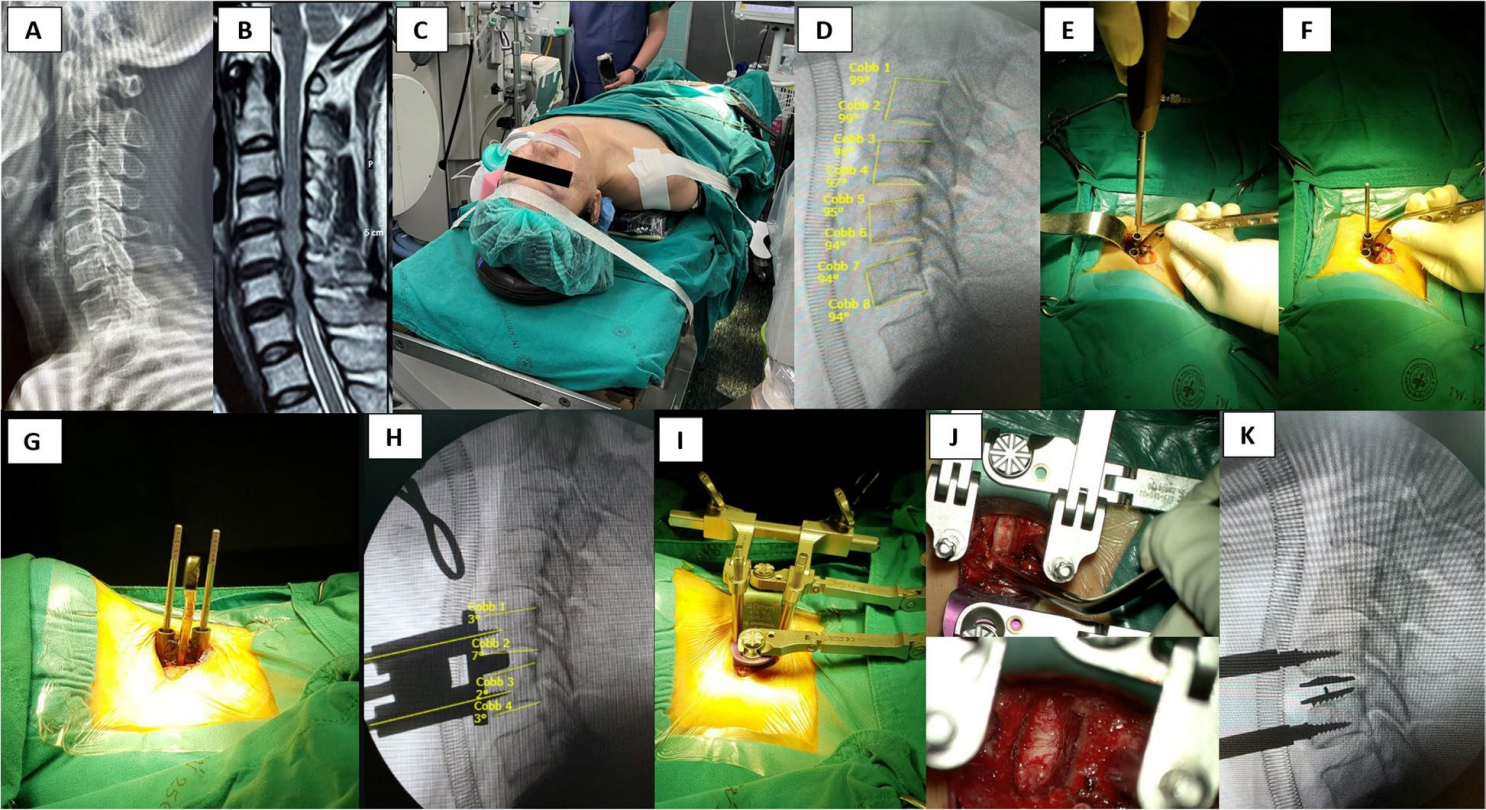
Patient 1 was performed Single-level C-TDR C5-6. Preoperative lateral X-ray (A), Sagittal MRI (B), Patient positioning (C), Intraoperative measurement of C5-C6 superior and inferior endplate angle (D), First Caspar pin placement using the aiming device (E, F), Second Caspar pin placement (G), C5-6 Caspar pin placement measuring SE-CP & IE-CP angles (H), C5-6 Caspar cervical distractor placement (I), Caspar cervical distractor system creating the working space (J), Intraoperative lateral fluoroscopic view (K)
